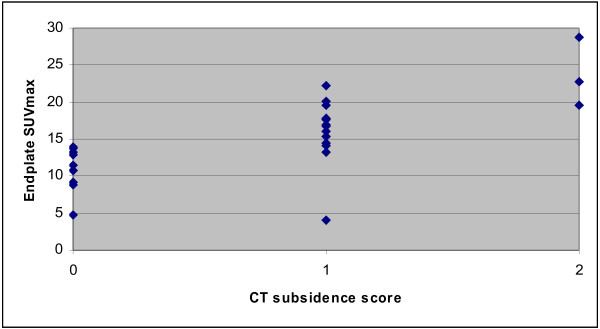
^18^F-fluoride activity in vertebral subsidence. SUVmax values of vertebral upper and lower endplates within corresponding subsidence scores on CT.
